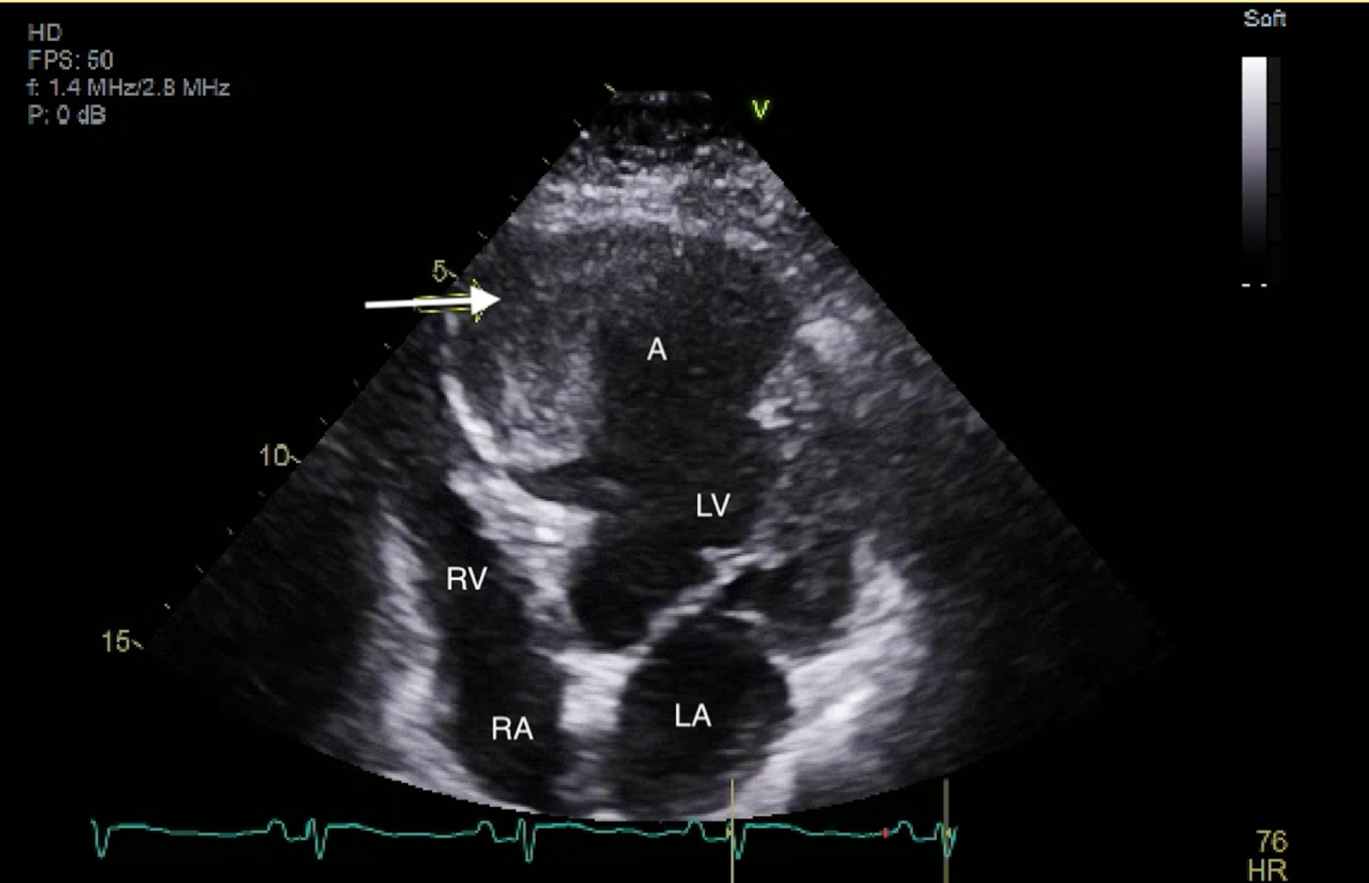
Ultrasonography confirmed aneurysmal dilatation of the left ventricle (arrow). RV - right ventricle; RA - right atrium; LV - left ventricle; LA - left atrium; A - aneurysm
